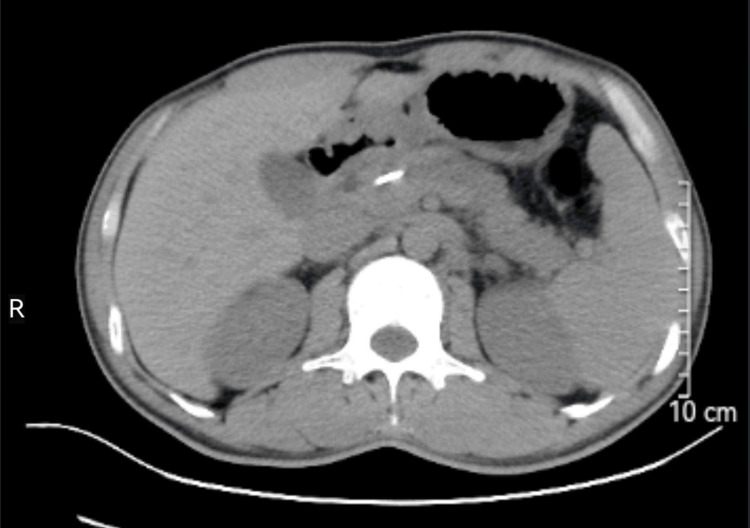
CT scan after four months post-initial procedure indicated the total disappearance of the cyst in the head and body of the pancreas.
